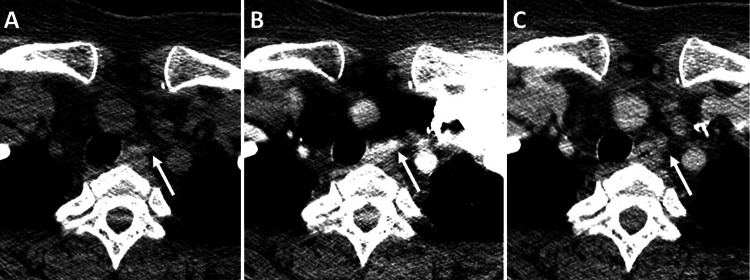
The 4D CT neck. (A) Non-contrast, (B) arterial phase, and (C) venous phase after the administration of 100 ml of intravenous contrast Iopamidol 76% (Isovue 370, Bracco) into the left upper extremity. Streak artifacts from the venous contrast limit accurate evaluation. However, there is a 6 mm soft tissue nodule in the superior mediastinum on the left ventral to the esophagus (white arrows). This nodule demonstrates suspected enhancement in the arterial phase with washout in the venous phase, raising concerns for a suspicious parathyroid adenoma.
